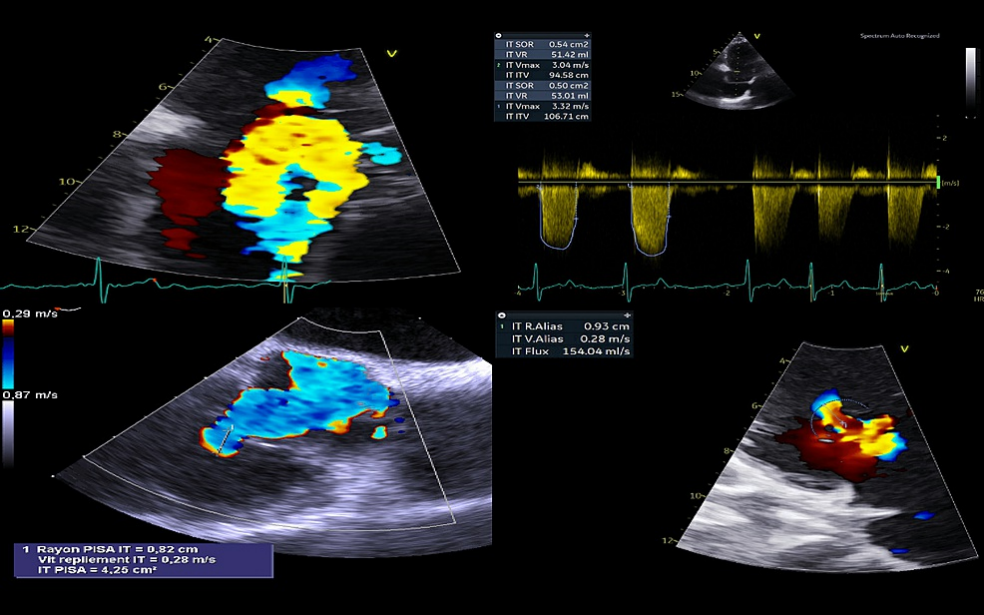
Pre-operative echocardiogram images showing severe tricuspid valve regurgitation
